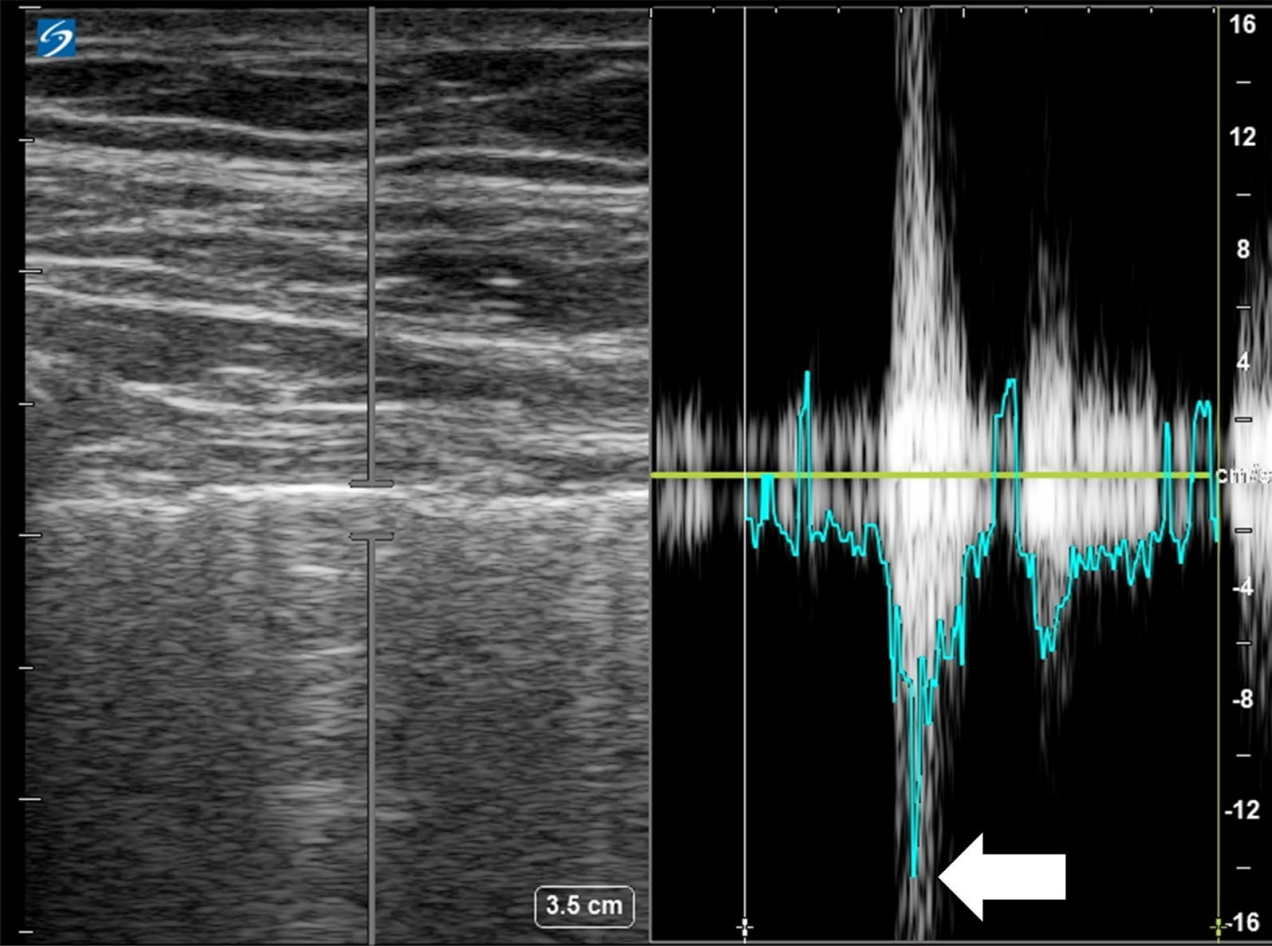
Example measurement of pleural velocity by pulsed wave Doppler. A maximum pleural velocity of 14.5 cm/s (white arrow) was measured with the Doppler reading gate positioned at the pleural line
